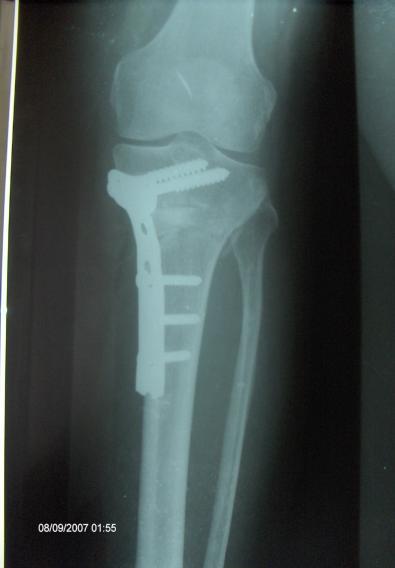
Radiographie du genou face objectivant une ostéotomie d'addition interne avec cale de ciment ostéosynthésée par plaque en L à 3 mois de recul
